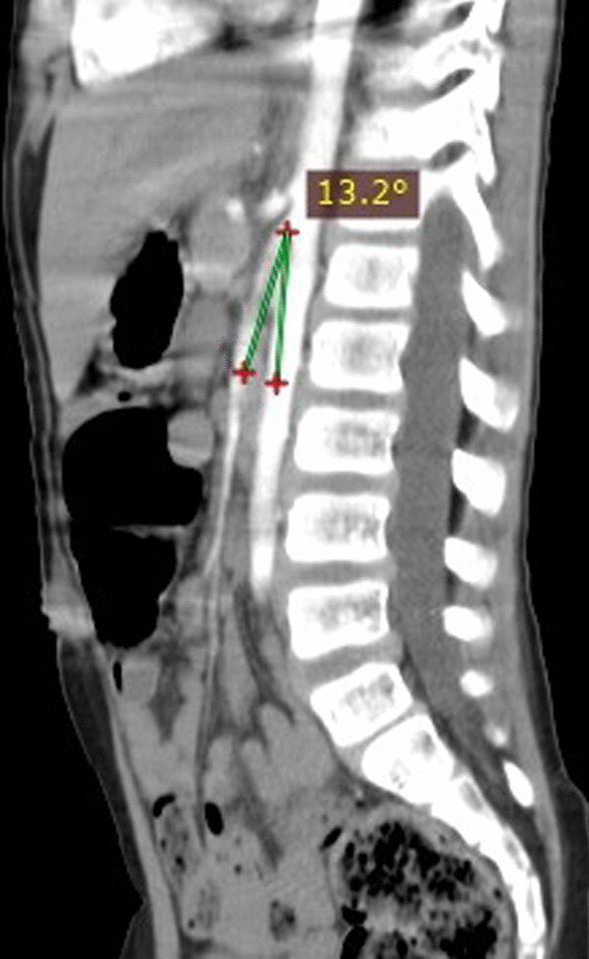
Contrast enhanced CT scan abdomen and pelvis (sagittal view) showing the angle between the abdominal aorta and the superior mesenteric artery
